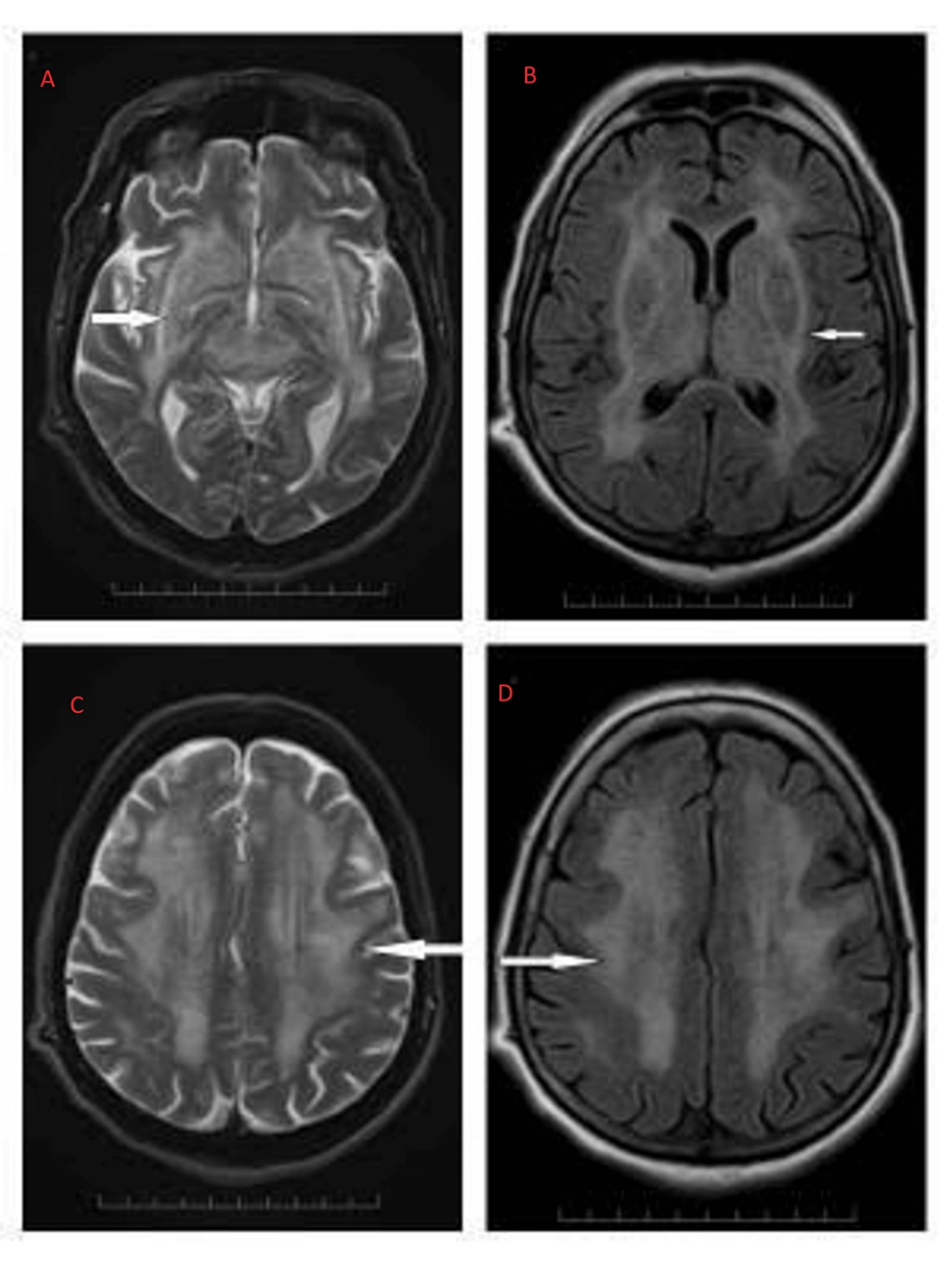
Brain MRI on admission: T2WI and FLAIR images in axial view showing bilateral symmetrical hyperintensities in BG and deep and subcortical white matter. Extensive confluent T2/FLAIR hyperintensities are noted. (A) The white matter surrounding BG is hyperintense on the T2WI sequence demarcating the lentiform nucleus from the surrounding structure. (B) FLAIR sequence also shows symmetrical hyperintensities involving BG. (C, D) T2/FLAIR images showing extensive deep and subcortical white matter involvement. MRI: magnetic resonance imaging; T2WI: T2-weighted imaging; FLAIR: fluid-attenuated inversion recovery; BG: basal ganglia.
